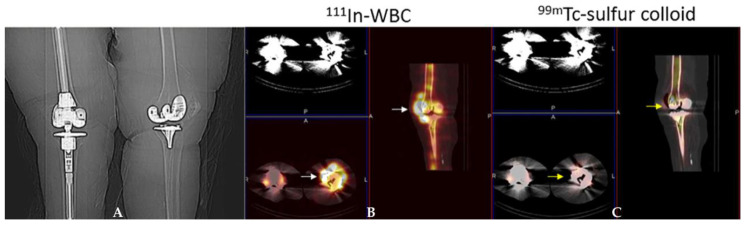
Periprosthetic infection left knee arthroplasty. Scout radiograph (A) demonstrates bilateral knee arthroplasties. The right is a revision arthroplasty and the left is a primary implant. On the ^111^In-WBC single photon emission computed tomography/computed tomography (SPECT)/CT) (B) there is abnormal labeled leukocyte activity along the anterior aspect of the left knee arthroplasty (white arrows). On the ^99m^Tc-sulfur colloid SPECT/CT (C), there is no corresponding activity in this region (yellow arrows) and therefore the study is positive for infection.
